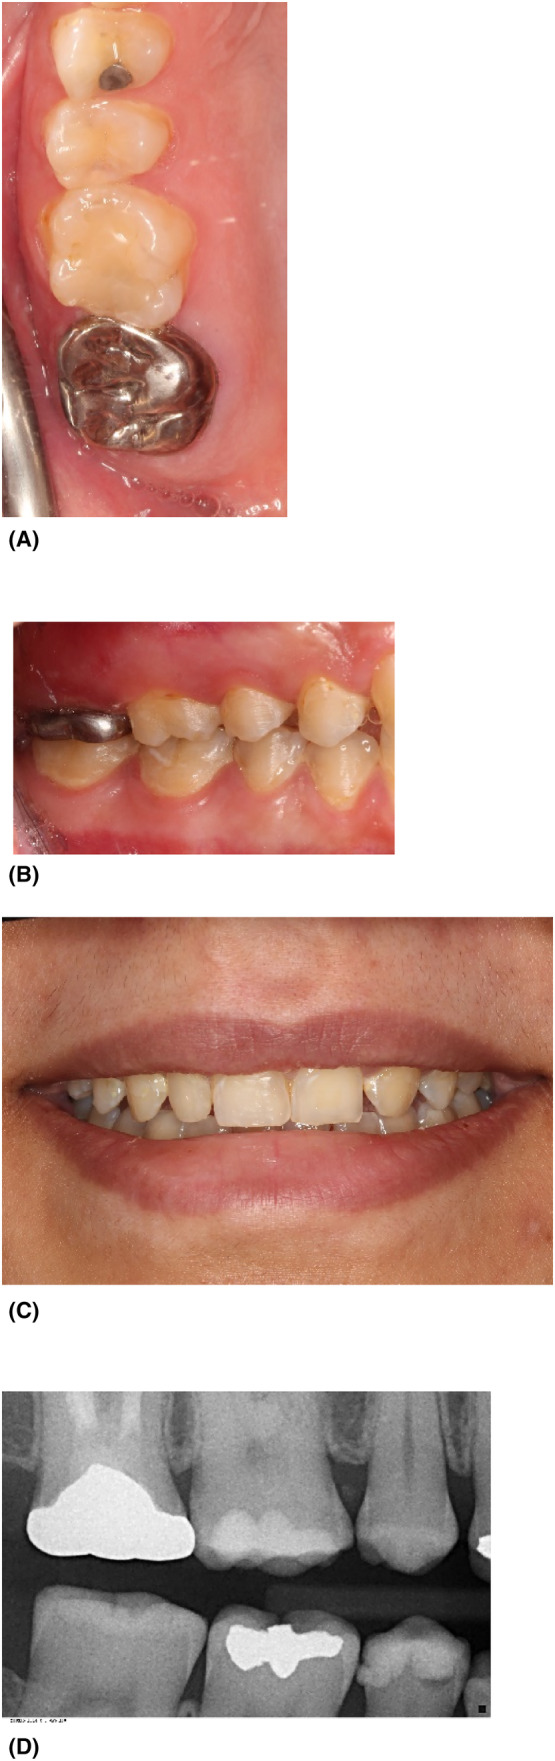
Final results (A), lateral view (B), patient smile (C), bitewing radiograph after cementation.
